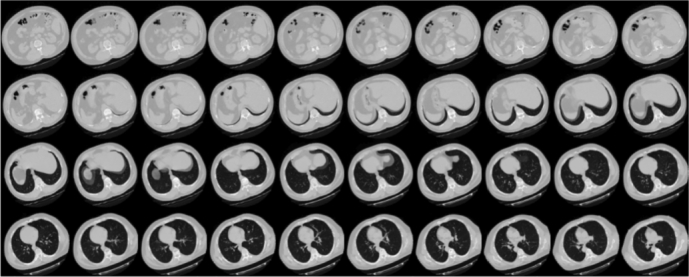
Volume data of slices of CT scans.
